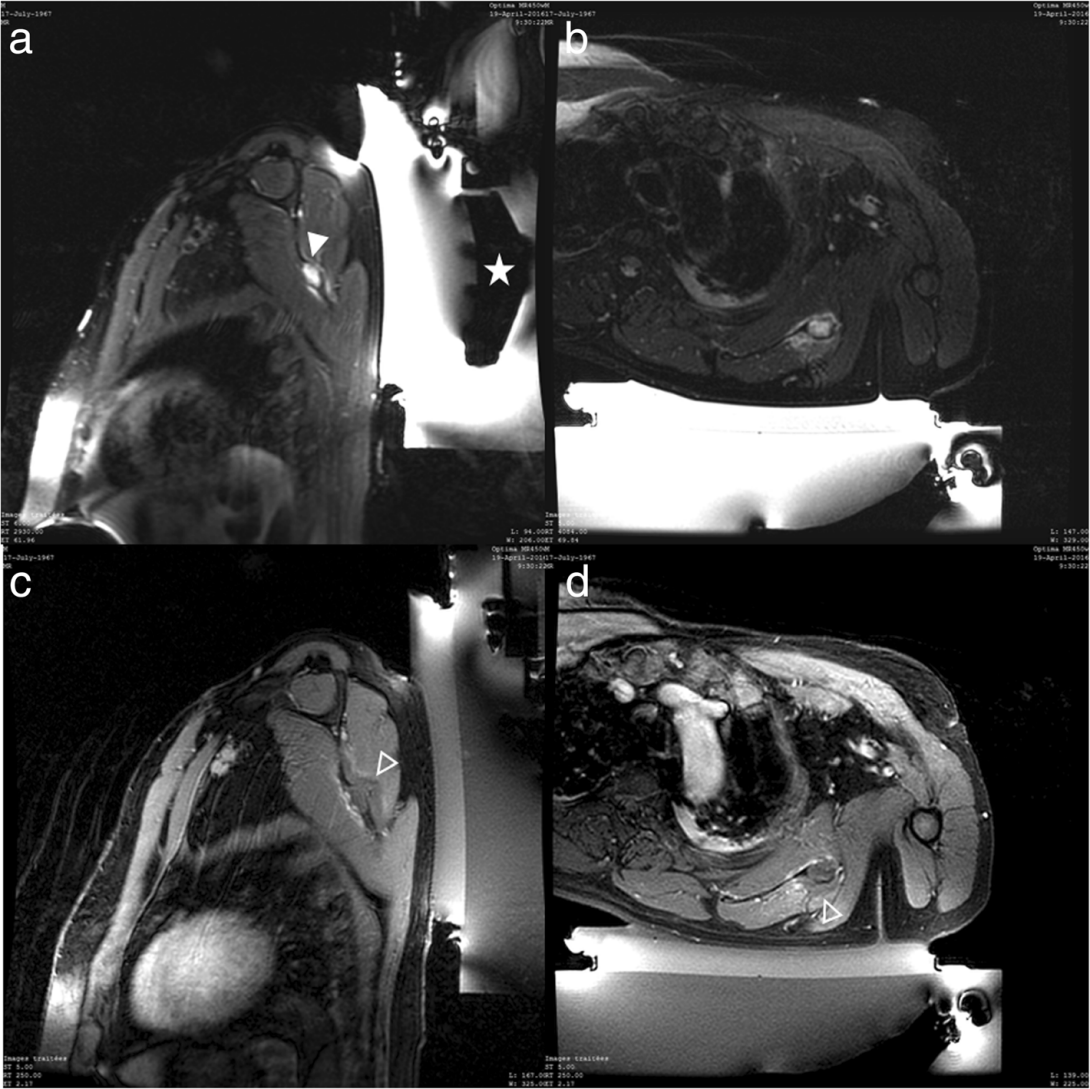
Patient 6. a Sagittal T2-weighted fat-suppressed MR images showing a bone metastasis of the scapula (arrowhead) in hypersignal in front of the transducer (star) before the procedure; b, c, d Axial T2, Sagittal and Axial T1-weighted fat-suppressed with contrast MR images after the procedure. The non-enhanced area (hollow arrowhead) is larger than the lesion and is clearly visible after the injection and corresponds to the zone of thermal destruction
